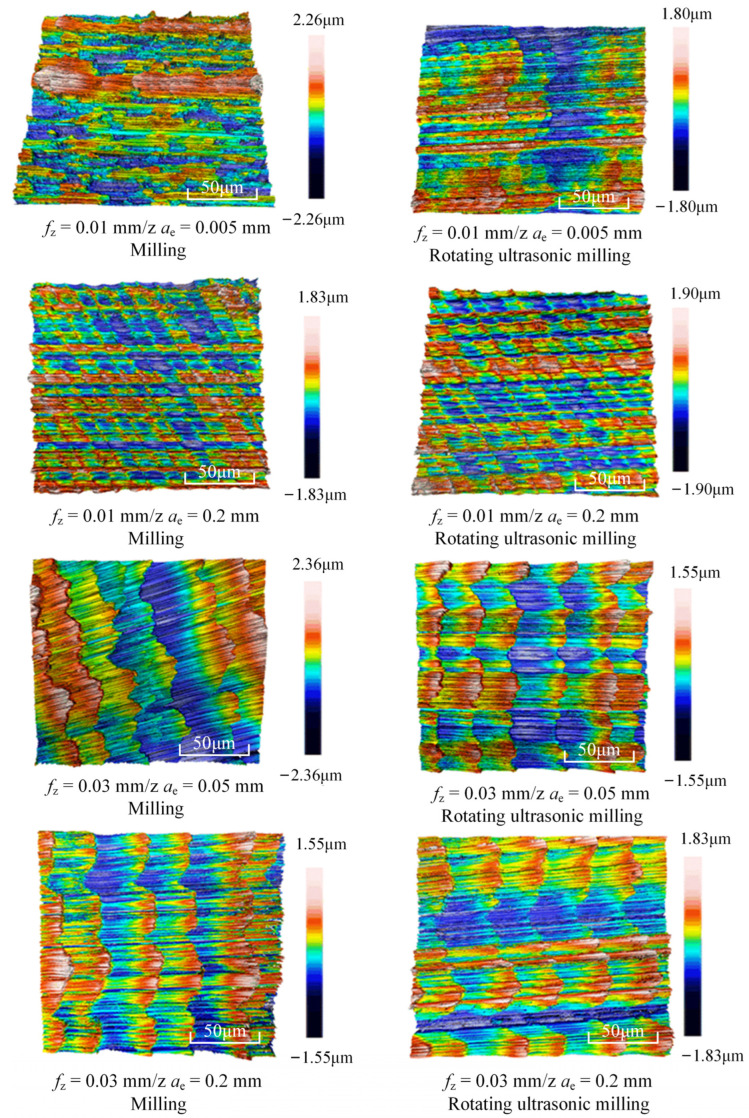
Three-dimensional topography of the machined surface by conventional flank milling and rotary ultrasonic flank milling.
